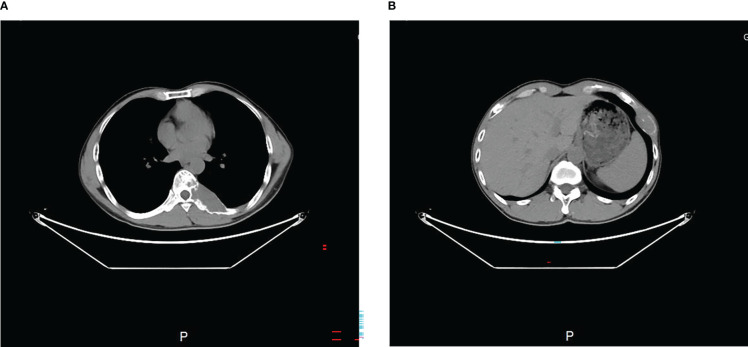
Preoperative transverse CT scan (A, B) showed the tumor occupied the 8th thoracic vertebral body and 7th rib.
